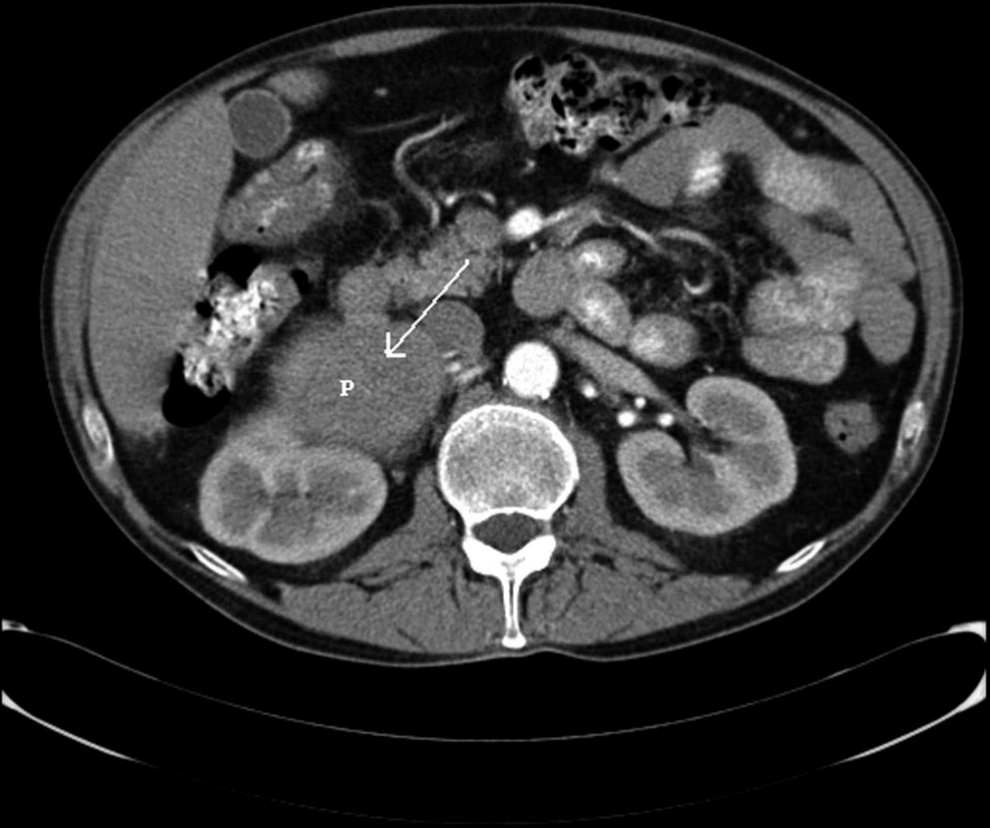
Abdominal computed tomographic scan showing a huge mass (*arrow*) starting from the right kidney
